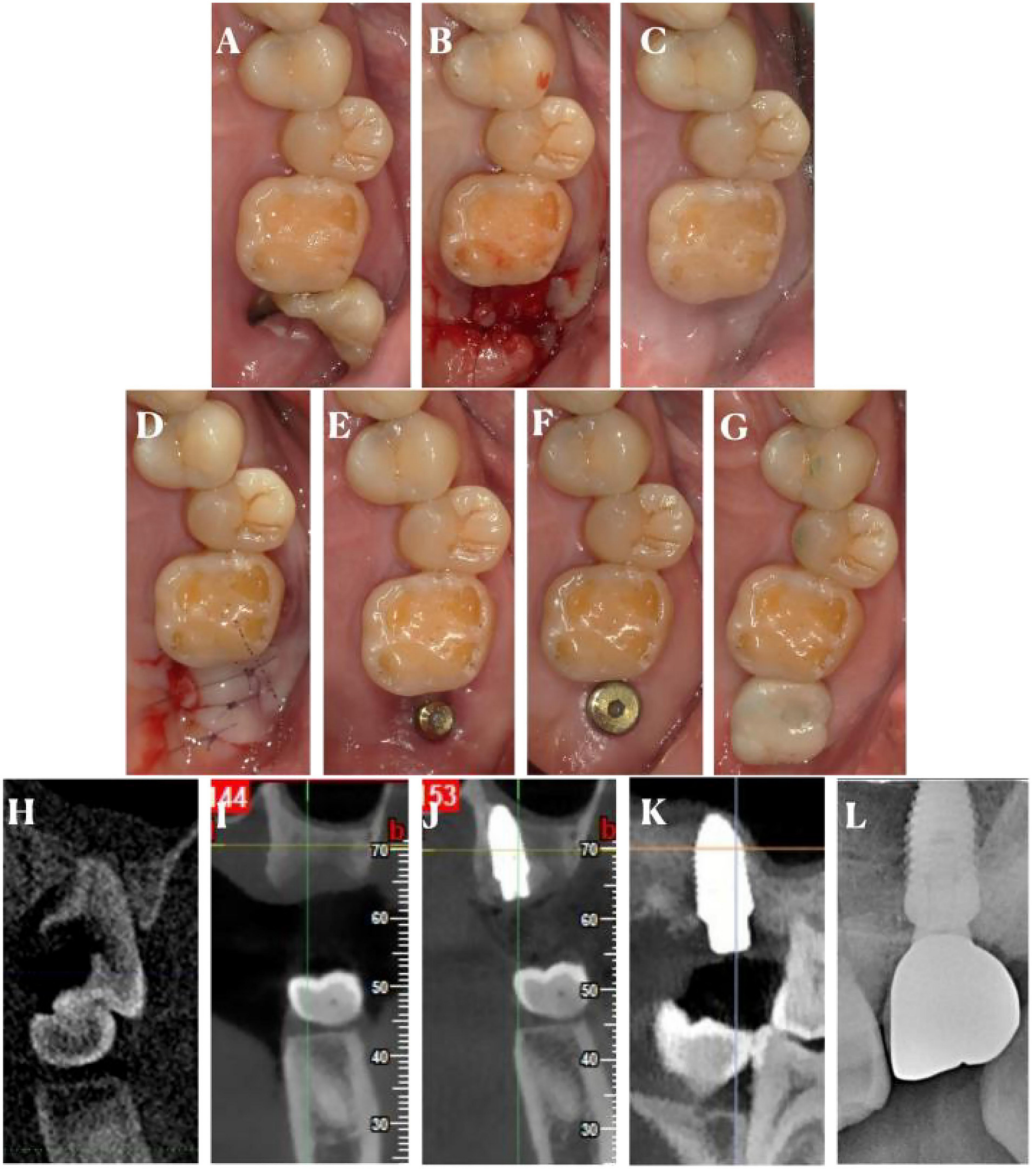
The procedures of the left side of #27. Intraoral photos (A), before tooth extraction; (B), after removed sutures; (C), before implant insertion; (D), after implant insertion; (E), before second stage operation; (F), after second stage operation; (G), after implant restoration; Radiograph (sagittal view of CBCT scan) (H), before tooth extraction; (I), before implant insertion; (J), after implant insertion; (K), before second stage operation; (L), after implant restoration.
